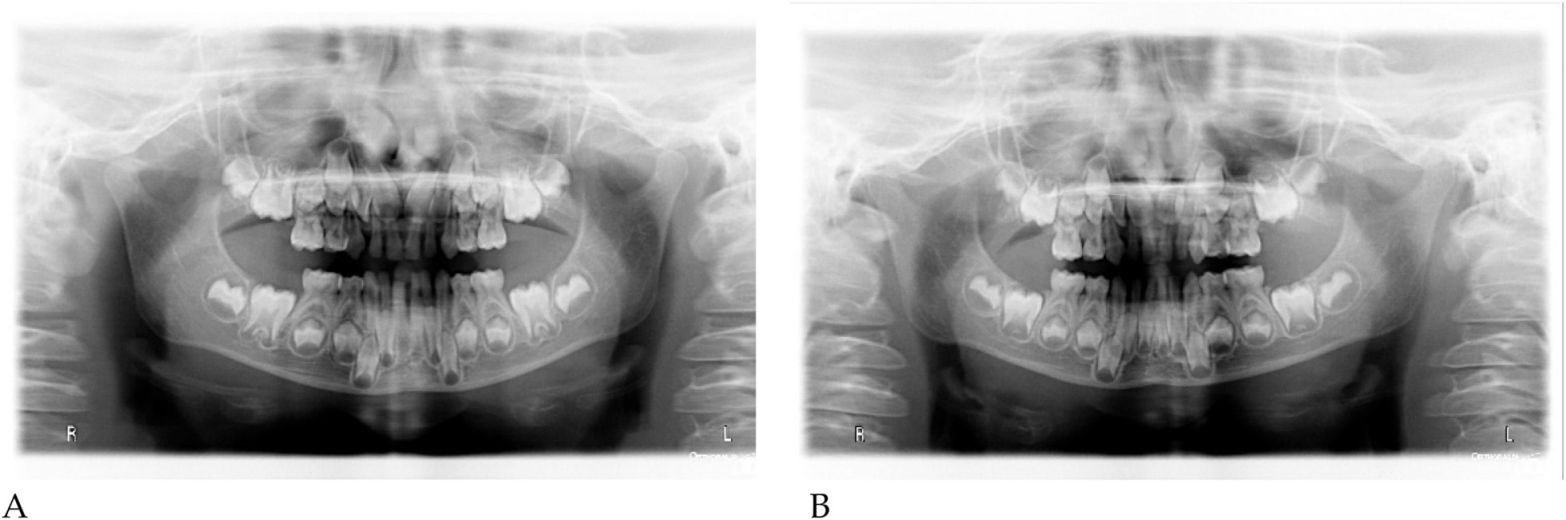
Pre- (A) and post-treatment (B) panoramic radiographs of case 9.
